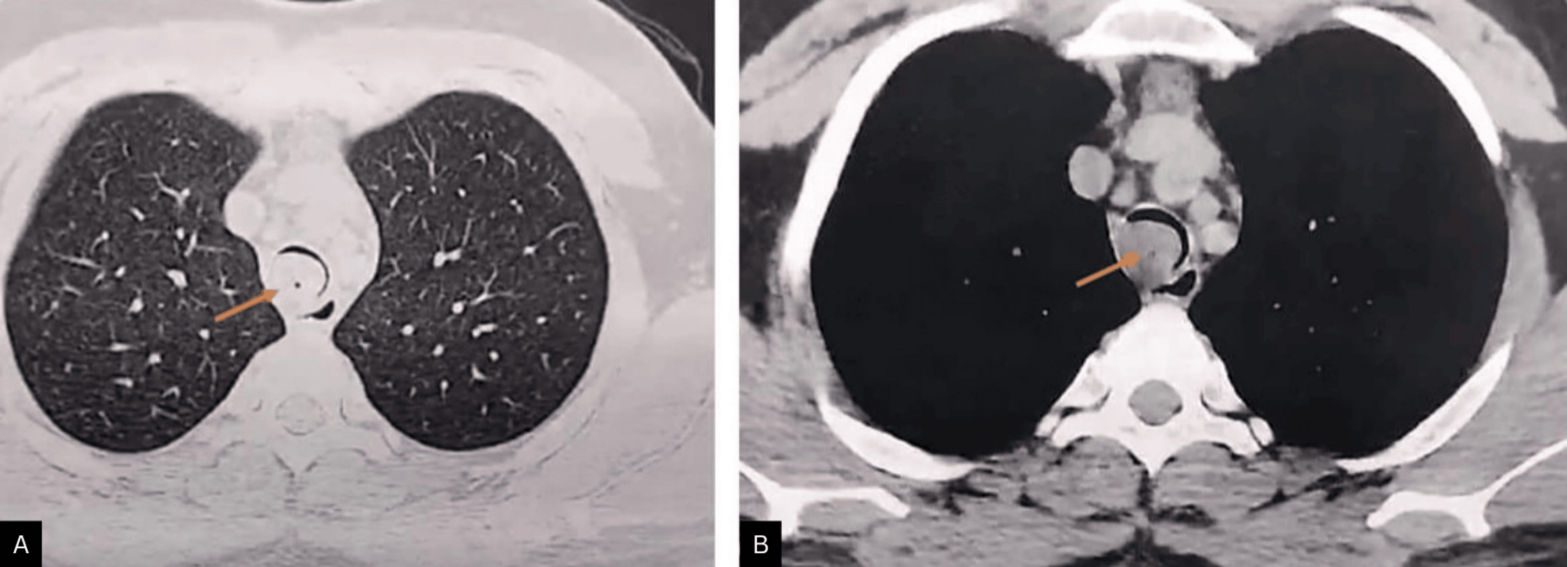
Axial HRCT chest images in lung (A) and mediastinal (B) windows demonstrate a well-defined endoluminal soft-tissue lesion arising from the mid-tracheal wall, causing near-complete luminal obstruction, consistent with a tracheal mass (arrows). HRCT: high-resolution computed tomography
